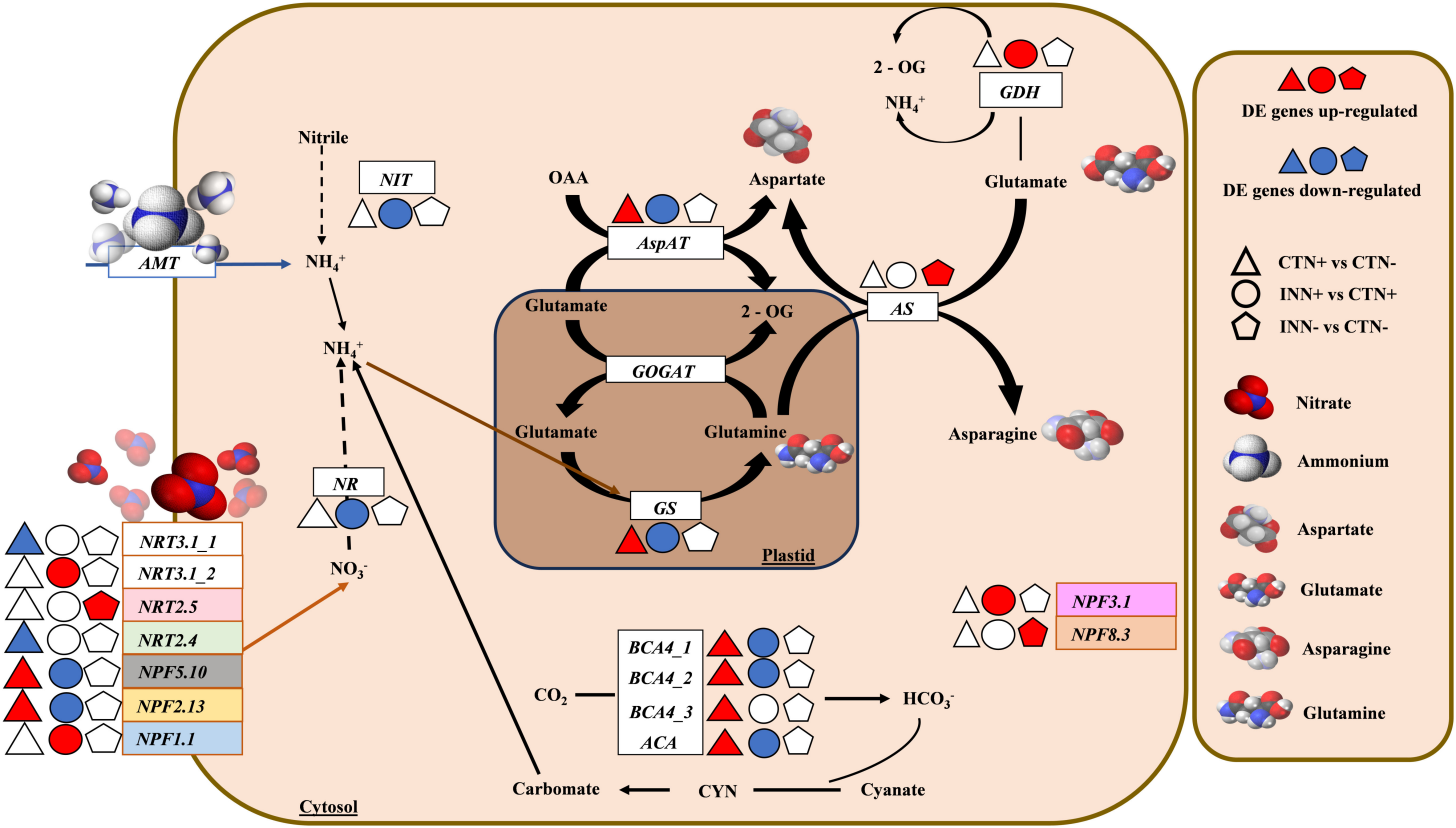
Overview of a scheme showing the regulation of nitrogen metabolism. This model presents the differentially expressed genes of maize plants inoculated with *H. seropedicae* ZAE94 (IN) or mock-inoculated (CT), and cultivated in 0.3 mM (N-) or 3 mM (N+) of nitrate. Up-regulated (red colors) or down-regulated (blue colors). White Symbol represents expressed genes, but not differentially regulated. *NIT*, nitrilase; *NRT*, nitrate transporter protein; *NPF*, nitrate transporter 1/peptide transporter family; *AMT*, ammonium transporter; *GOGAT*, glutamate synthase; *GS*, glutamine synthetase; *NR*, nitrate reductase; *BCA*, beta carbonic anhydrase; *ACA*, alpha carbonic anhydrase; *AspAT*, aspartate aminotransferase; *AS*, asparagine synthase; *GDH*, glutamate dehydrogenase; *CYN*, cyanate hydratase; *2-OG*, 2-oxoglutarate; *OAA*, oxaloacetate.
